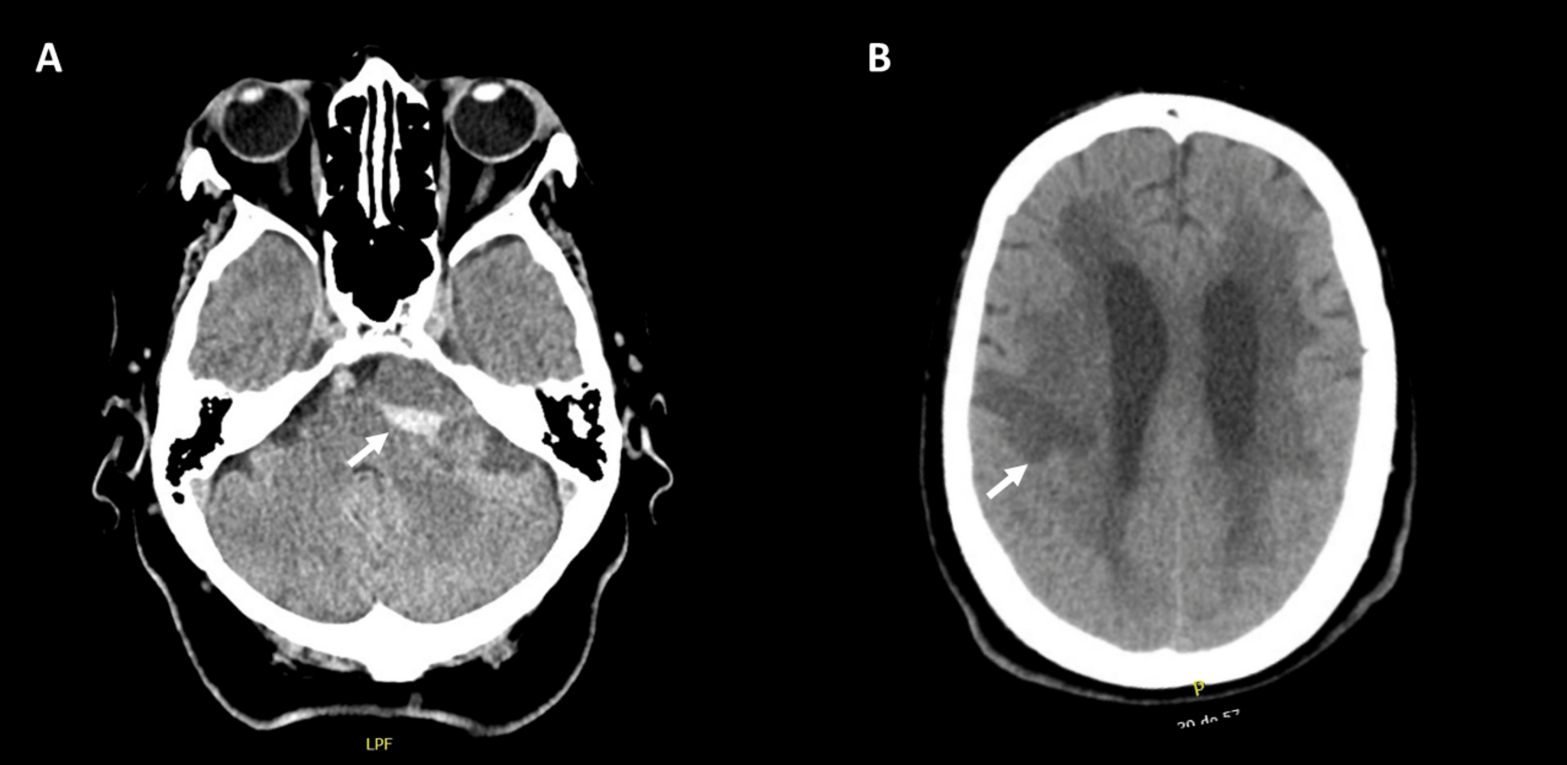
(A,B) Axial cranial CT documenting increased hematic density of the space-occupying lesion (arrow in A), without obstruction of the ventricular system, and recent ischemic lesion in the partial territory of the right middle cerebral artery (arrow in B).
